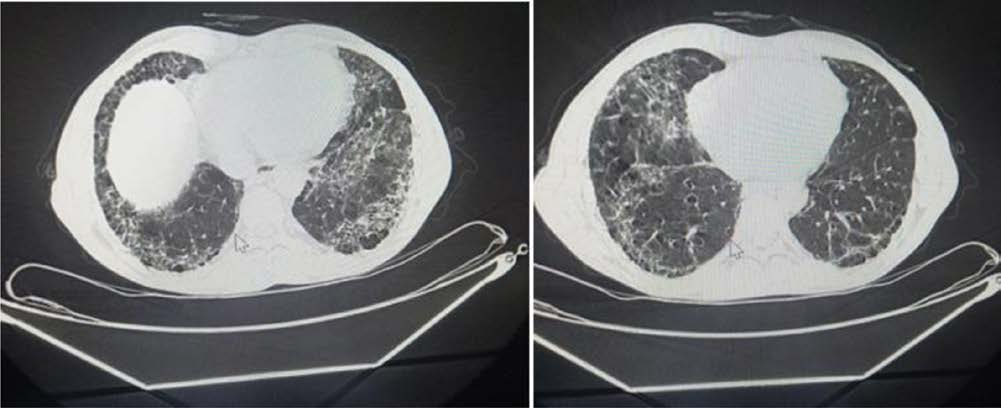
A noncontrast multisegment CT scan of the chest demonstrated reticular densities with honeycomb patterns distributed throughout the lung areas, particularly at the bases and peripheries. CT = computed tomography.
